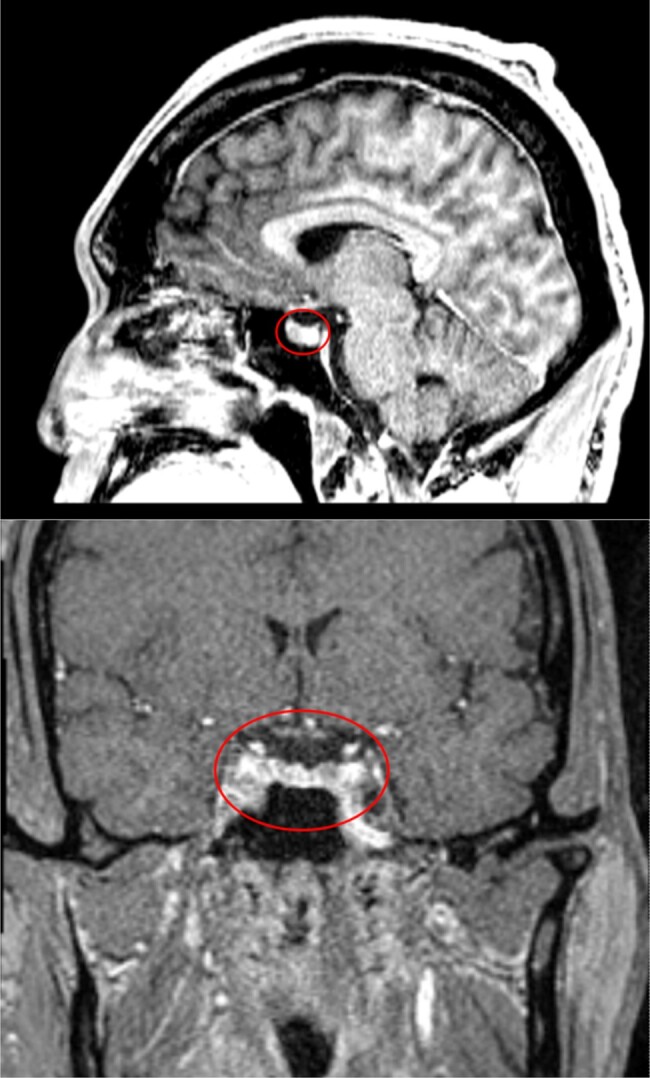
MRI of the pituitary (coronal and sagittal views) showing 10-mm macroadenoma, marked by red circle. Frontal bossing of cranium can be seen to the left of the sagittal image.
